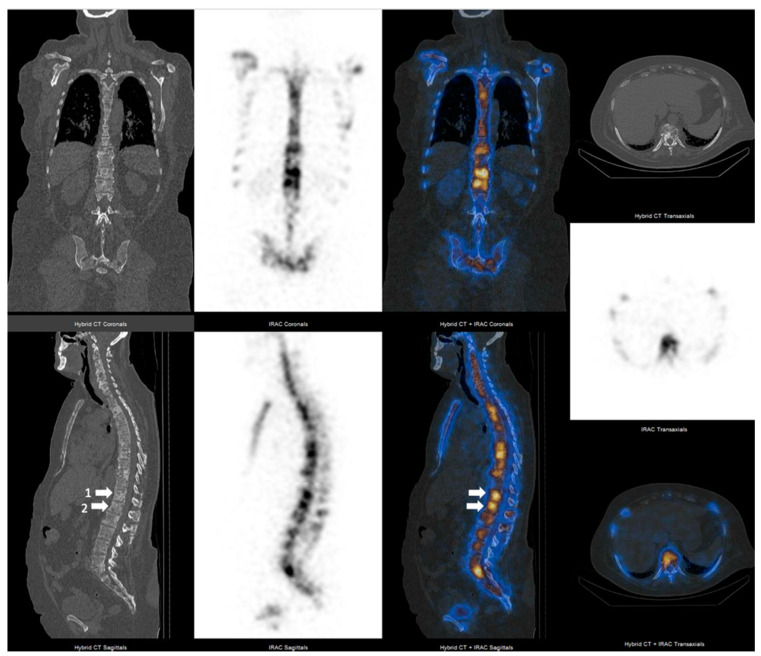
62 year-old breast cancer female patient with grade 2 ductal invasive carcinoma presenting with pain and elevated alkaline phosphatase, with high radiotracer uptake in multiple lytic bone metastatic lesions (1–2 = lytic lesions with the highest SUVmax value localized in T12-L1 vertebrae).
